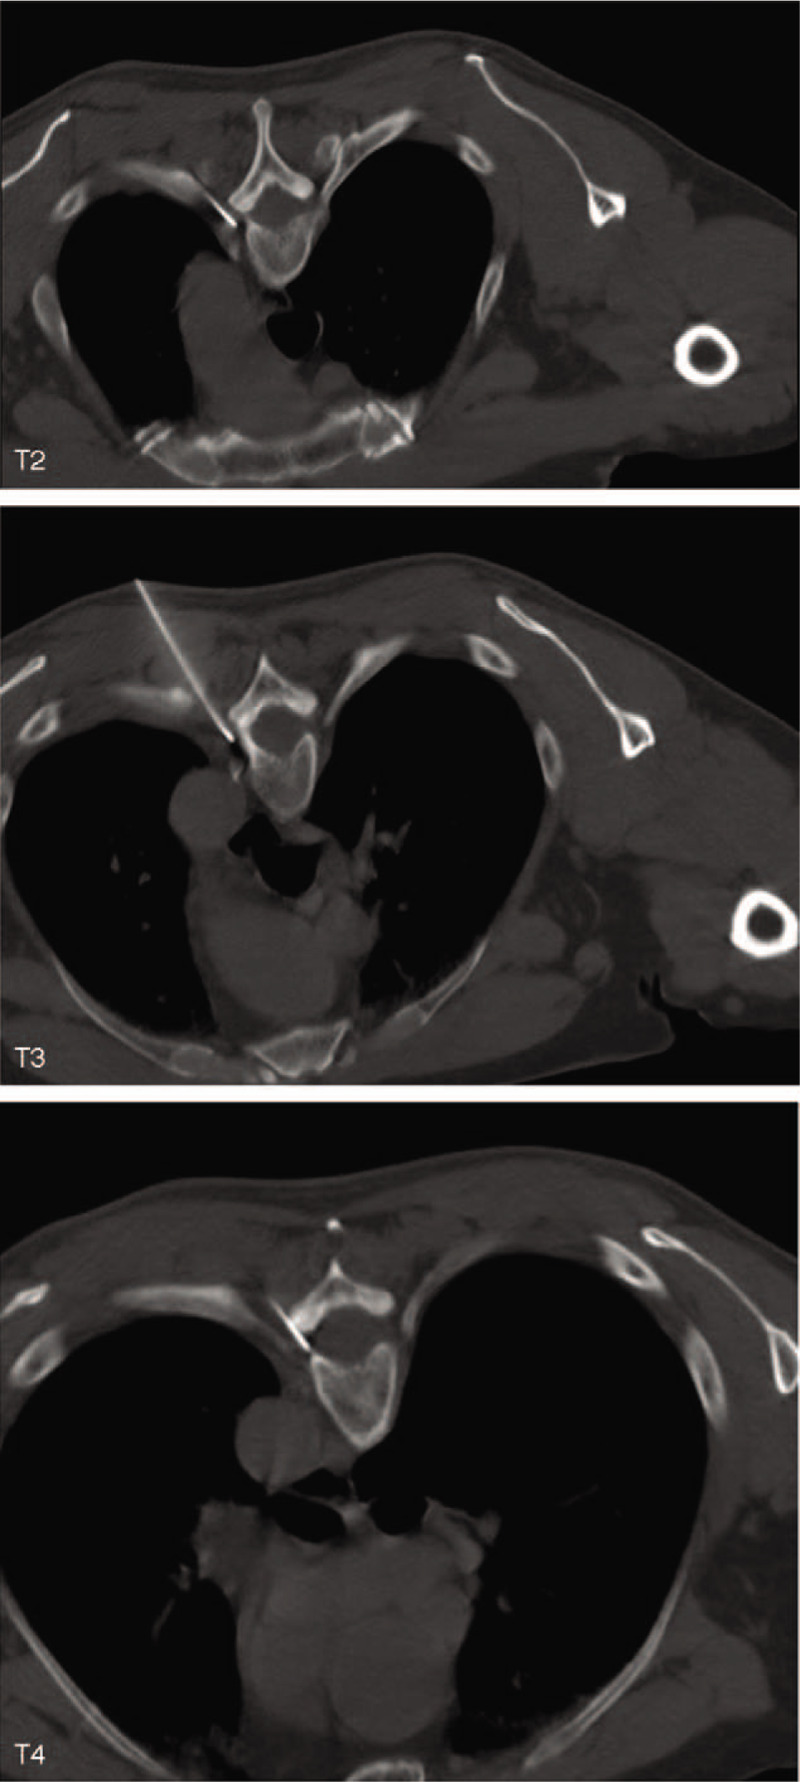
CT-guided puncture of left T2, T3, and T4 dorsal root ganglion to 1/3 of the intervertebral foramen. CT = computed tomography.
